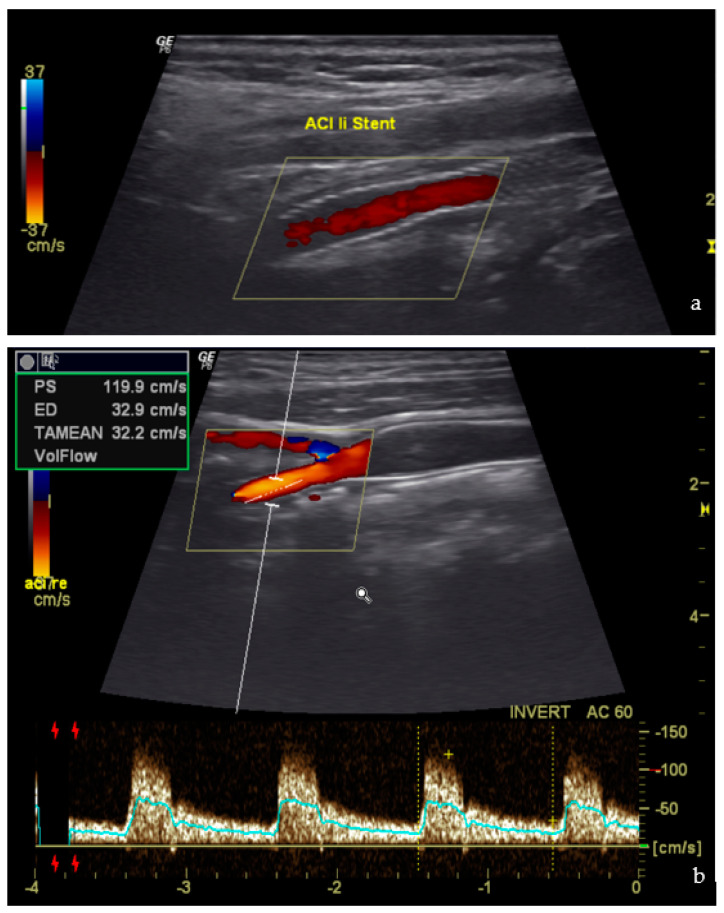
Postoperative ultrasound (a) Duplex of the Stent course (b) Doppler of the ICA.
